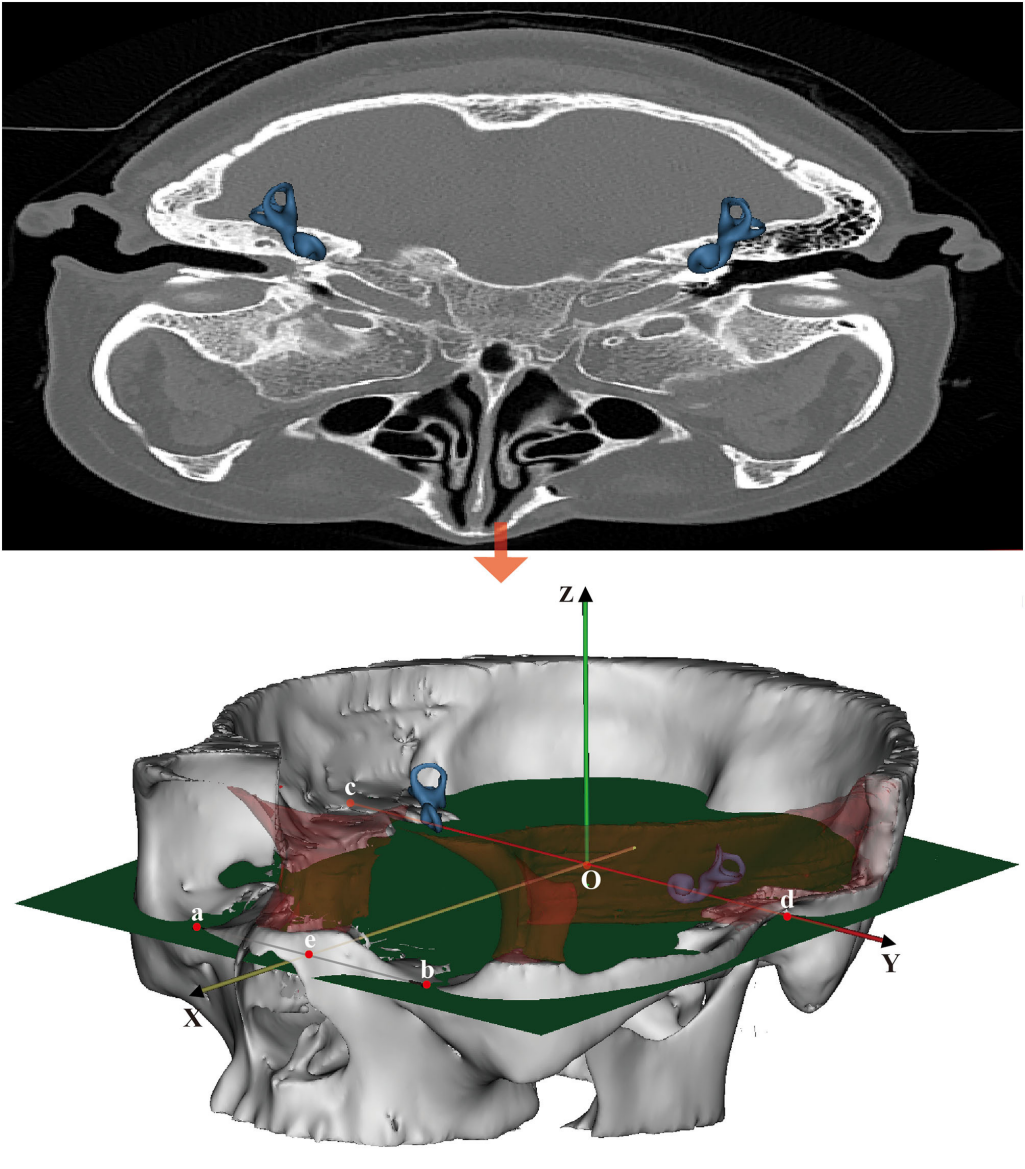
Reid's coordinate system overlaid on a CT image. Points a and b are the bilateral midpoints of the inferior margin of the orbits. Points c and d are the bilateral midpoints of the external auditory canal. Point e is the midpoint of the line segment $\bar{\text{ab}}$. The origin of the coordinate system, point O, is the midpoint of line segment $\bar{\text{cd}}$. The *x-* and *y-*axes are defined by the rays $\vec{\text{Oe}}$ and $\vec{\text{Od}}$, respectively, and the *z*-axis is perpendicular to *x* and *y* (+superior). The sagittal plane (SP) was defined as the plane that contains point O and is perpendicular to the *y*-axis.
